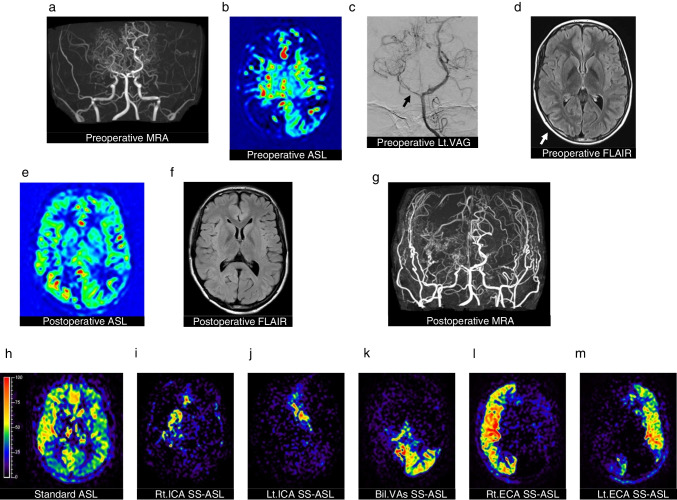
(a) Preoperative magnetic resonance angiography (MRA) demonstrating multiple changes in arterial vascular calibers and (b) Preoperative arterial spin labeling (ASL) showing reduced cerebral blood flow. (c) Preoperative left (Lt.) vertebral angiography (VAG) showing right posterior cerebral artery (PCA) stenosis (arrow) and (d) Preoperative Fluid-attenuated inversion recovery (FLAIR) showing ivy sign (arrow). (e) Postoperative ASL showing improved blood flow in the whole brain and (f) Postoperative FLAIR showing disappearance of ivy sign and no abnormal findings. (g) MRA showing excellent development of the bypasses from the bilateral external carotid artery (ECA). (h) Standard ASL showing improved blood flow in the whole brain (in ml/100 g/min). (i, j) superselective-ASL (SS-ASL) showing that each internal carotid artery (ICA) supplies the ipsilateral basal ganglia, (k) bilateral vertebral arteries (VAs) supply the left occipital lobe, (l) the right ECA supplies cortical areas of the anterior and posterior circulation in the right cerebral hemisphere and (m) the left ECA supplies cortical areas of the left anterior circulation including the right posterior circulation
